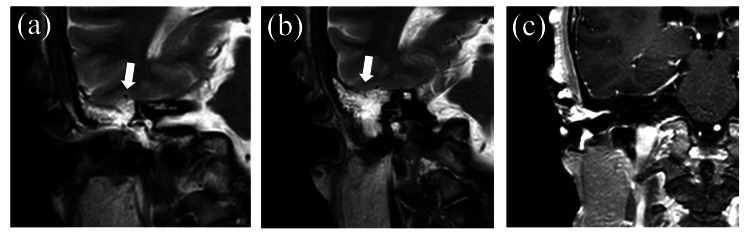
Preoperative magnetic resonance imaging (MRI) findings before the initial surgery (a) T2-weighted image showing hyperintensity in the region of computed tomography (CT) shading corresponding to the first fistula, (b) T2-weighted image showing hyperintensity in the region of CT shading corresponding to the second fistula, (c) no evidence of meningoencephalocele or arachnoid granulations on gadolinium-enhanced MRI.
